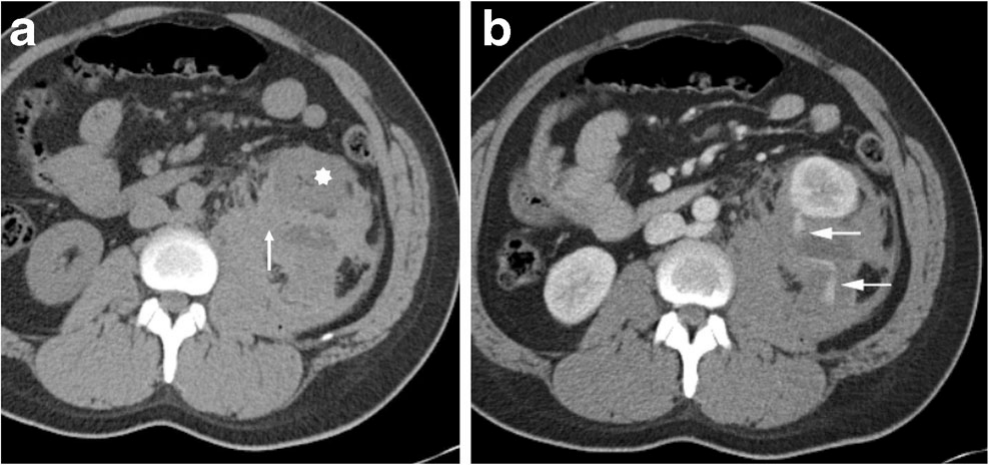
a Acute left-sided haematoma that is isodense to muscle (*arrow*), displacing the kidney anteriorly (*star*). b The portal venous phase shows active bleeding (*arrows*) into the haematoma. The bleed was secondary to an ultrasound-guided biopsy
